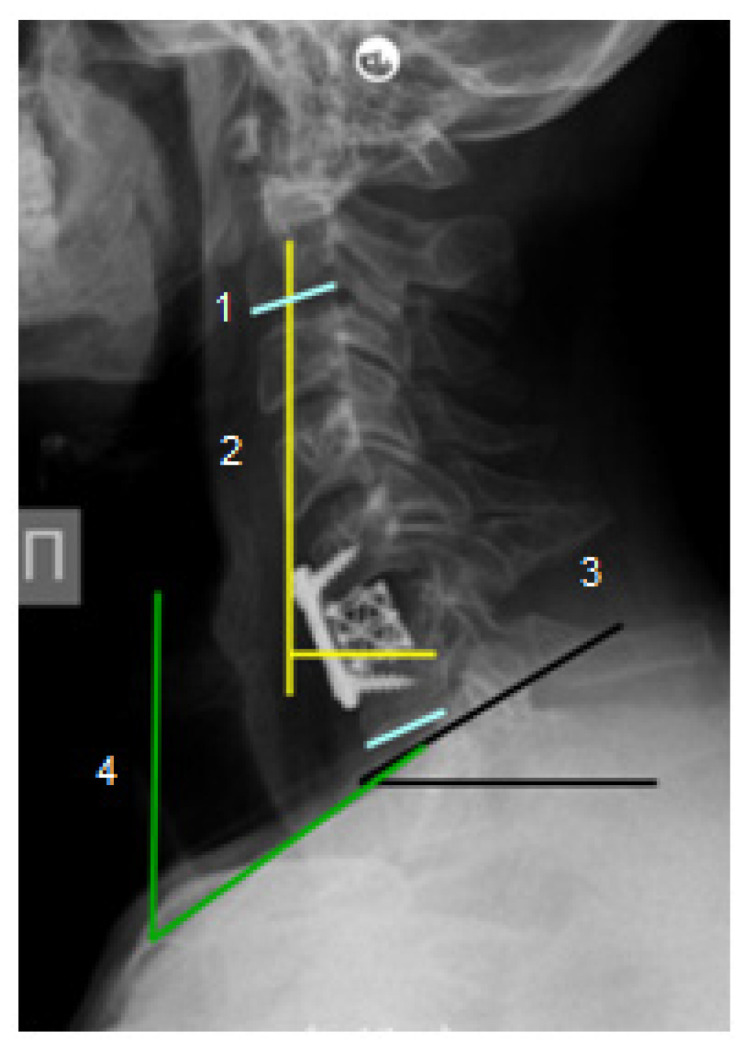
Cervical spine radiograph in the lateral projection in a patient with infectious cervical spondylitis. Symbols: 1—cervical lordosis C2-C7; 2—cervical sagittal vertical axis (CSVA); 3—Th1 slope (T1S); 4—neck tilt angle (NTA).
